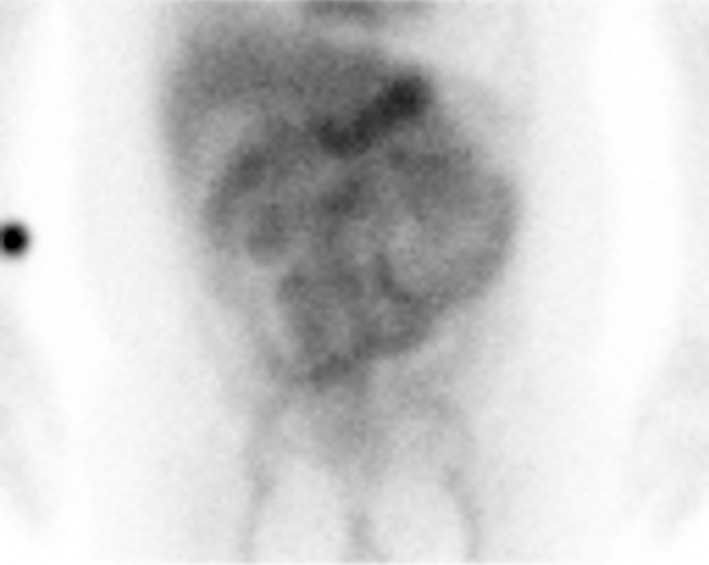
Technetium‐99 m‐labelled (^99m^Tc‐labelled) human serum albumin (HSA) scintigraphy. 2 minutes after intravaenous injection of ^99m^Tc‐labelled HSA, there was mild accumulation in the small bowel, and it became clear. 3 hours and 30 minutes after injection, there was movement of accumulation in the anus side. Typical features of protein losing gastroenteropathy were documented
